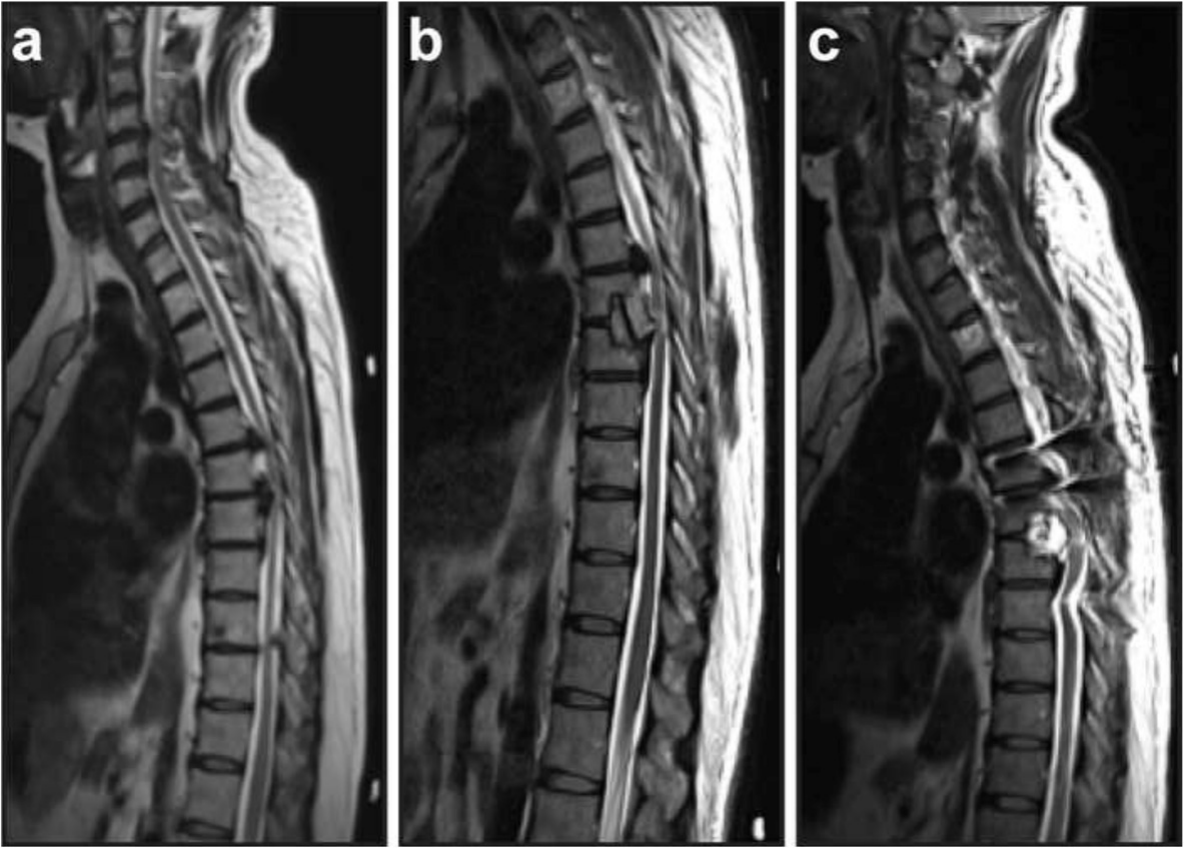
Magnetic resonance imaging (MRI) of the thoracic spine (T2). a The preoperative MRI (04/09/2015) b 1st MRI scan post-surgery (first surgery - 14/05/2015), c Post- operative MRI after the second surgery (second surgery - 20/04/2015)
